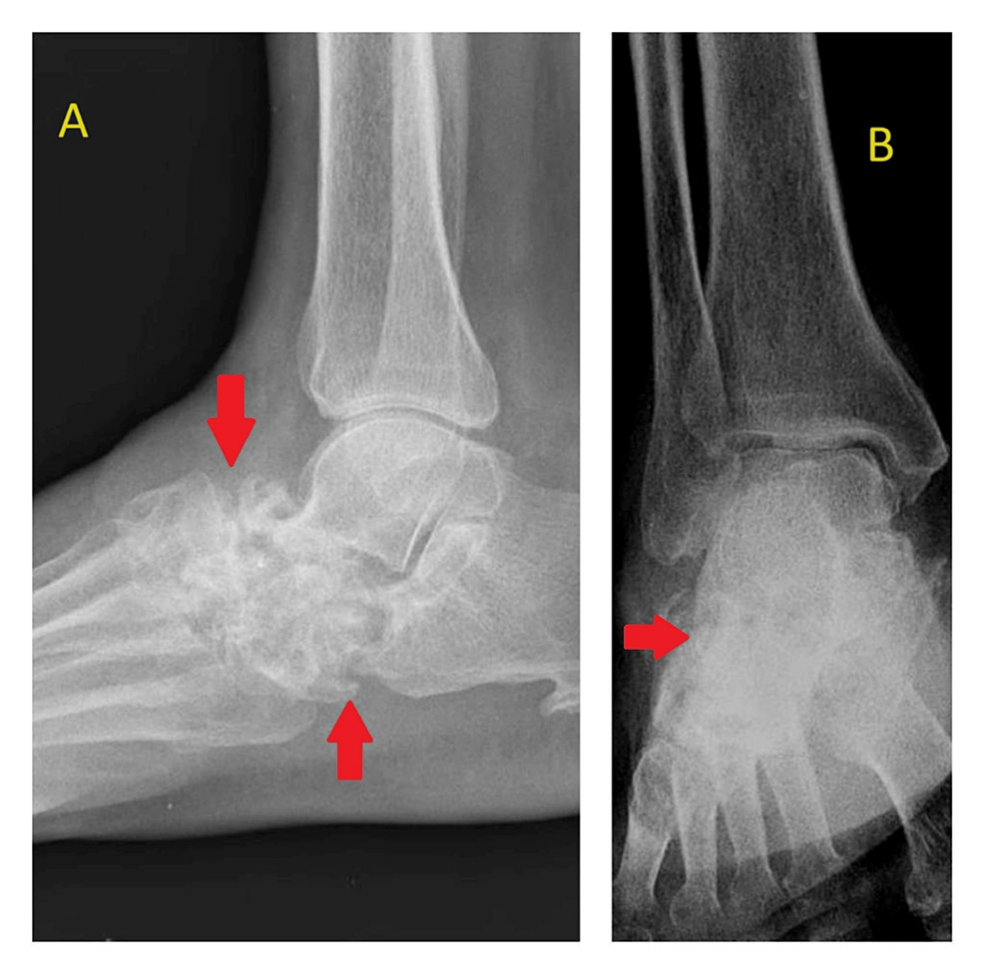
X-ray of right ankle lateral (A) and mortise (B) views showing only lytic lesions noted in the tarsal bones. No other osteomyelitis changes were seen.
